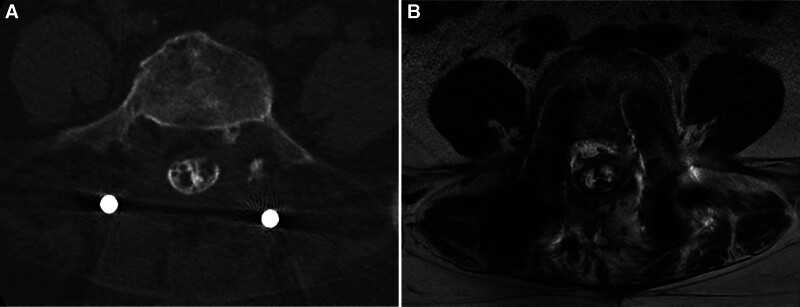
Axial CT imaging and Axial T2-weighted MRI of the ossification outcome: (A) demonstrates the CT of a characteristic honeycomb appearance of ossified areas, with calcified deposits penetrating the dural sac on postoperative month 25. (B) shows the MRI of low-signal intensity thickening in the dural sac and intradural regions, consistent with calcification and ossification on postoperative month 25. CT = computed tomography, MRI = magnetic resonance imaging.
